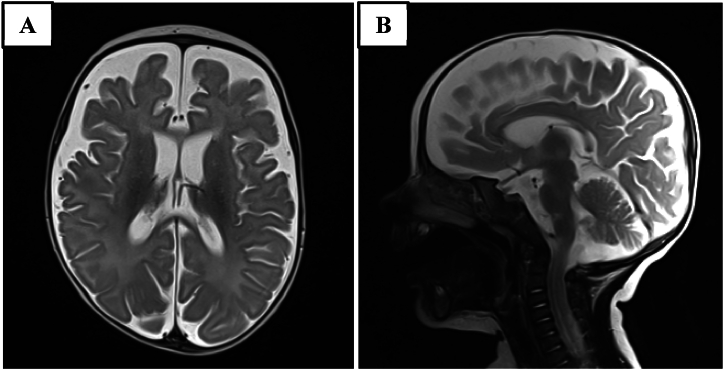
Brain MRI at admission. A. T2 TSE axial sequence. B. T2 TSE sagittal sequence.
